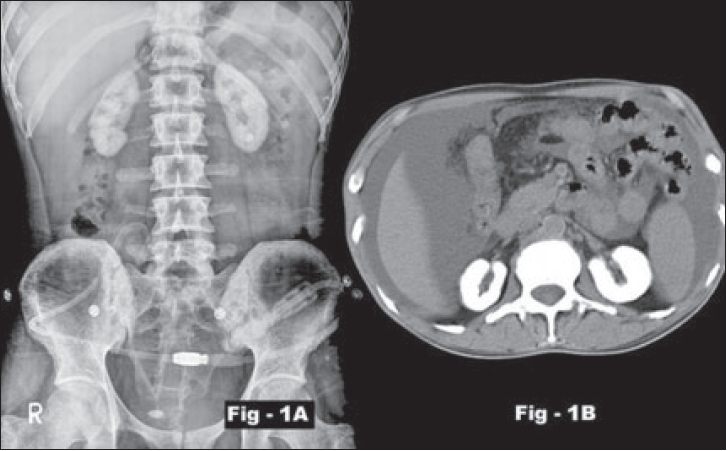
Plain X-ray KUB region (Fig. 1 A) and noncontrast CT abdomen (Fig. 1B) showing calcified kidneys
